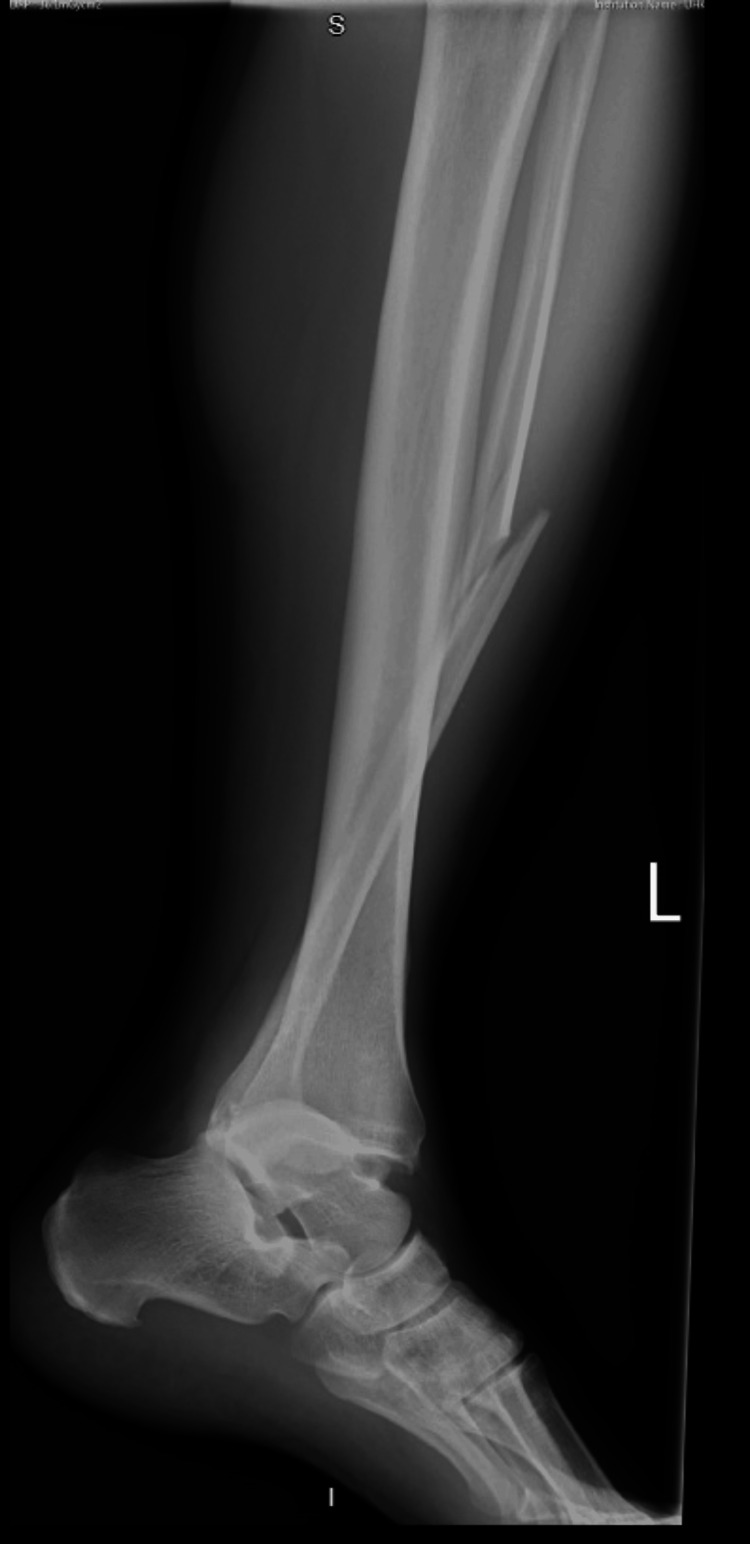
Lateral left ankle radiograph post-tackle playing football
